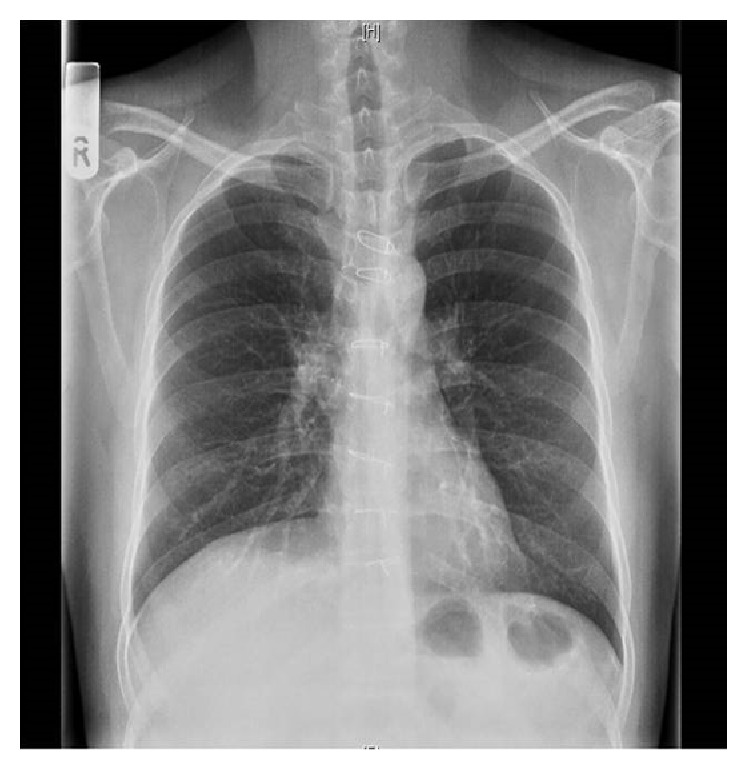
No residual lesion on chest X-ray on follow-up.
